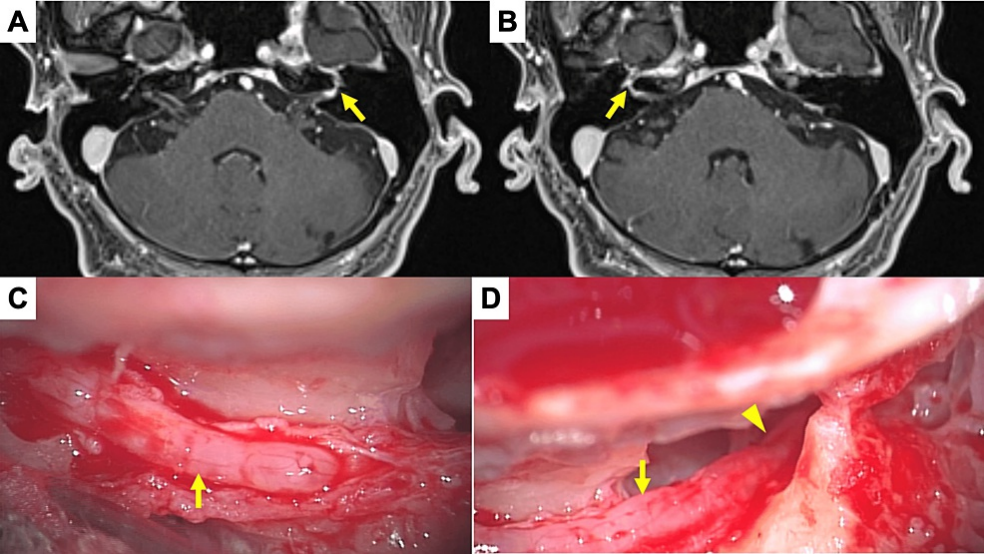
Contrast-enhanced MRI revealed enhancements of bilateral facial nerves with higher signal intensity on the left side and significant edema of facial nerve was observed during surgery A. An axial image of the labyrinthine and geniculate portion of the left facial nerve. The arrow indicates enhanced area. B. An axial image of the labyrinthine and geniculate portion of the right facial nerve. The arrow indicates enhanced area. C. Findings of vertical portion of the left facial nerve after decompression. The arrow indicates the edema of the nerve after cutting the sheath. D. Findings of horizontal-second geniculate portion of the left facial nerve after decompression. The arrow and the arrow head indicate the edematous nerve of second geniculate portion and horizontal portion after cutting the sheath, respectively.
